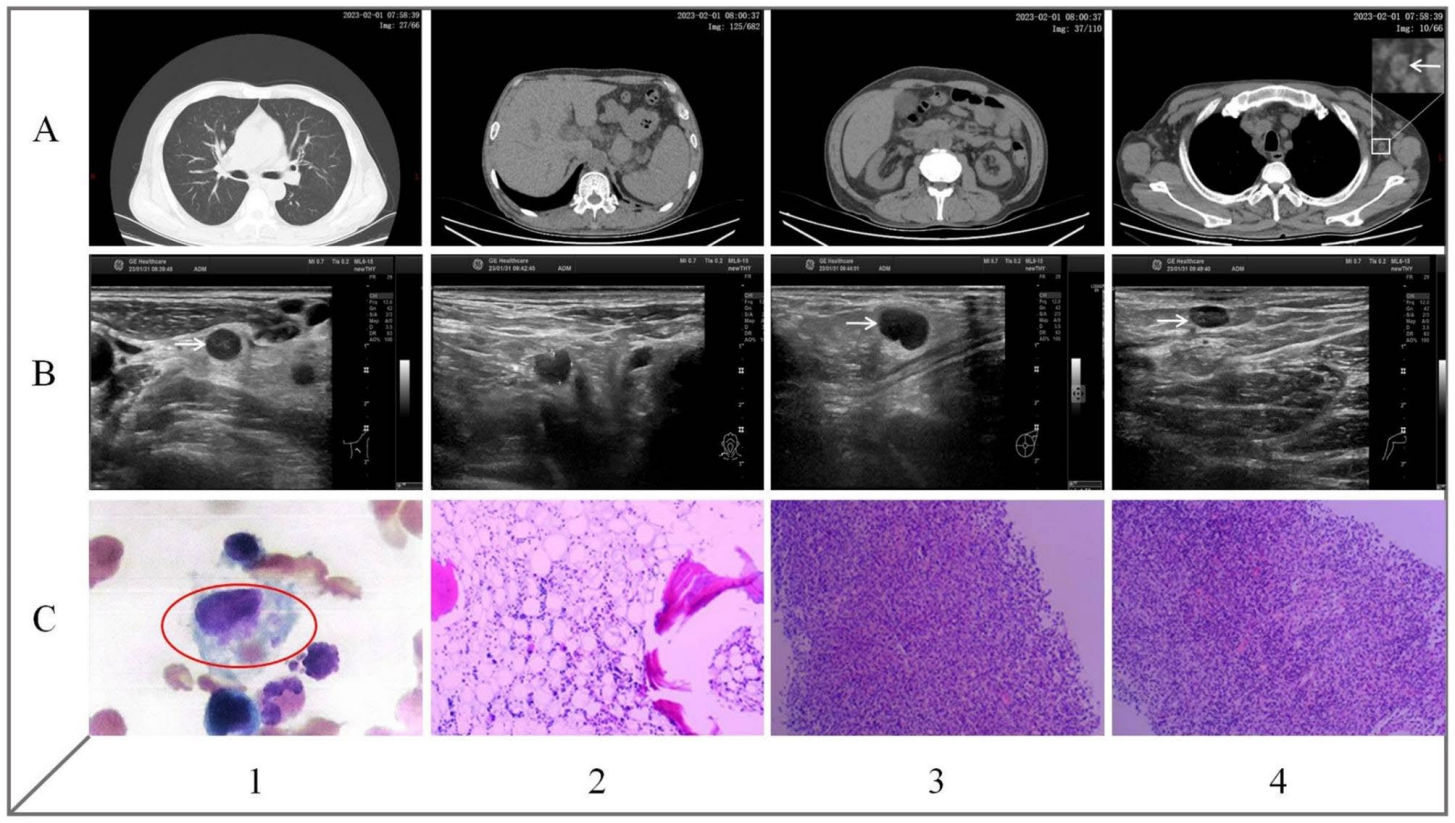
Imaging and morphologic findings of the index patient. A1-A3: Pulmonary, abdominal and pelvic CT scan shows no sign of infection, absence of hepatosplenomegaly and kidneys with slightly diminished size. A4: Mediastinal window depicts enlarged left axillary lymph node, with the ring-shape nodal necrosis (arrow). B1-B4: US image obtained at the same time as the CT scan shows a somewhat matted, hypoechoic nodal mass at the cervical, supraclavicular, axillary and inguinal sites, with an irregular perinodal border (arrow), suggesting perinodal infiltrates. C1-C2: Bone marrow aspiration and biopsy reveal trilineage hematopoiesis with hemophagocytosis (red circle). C3-C4: Lymph node biopsy finds that left cervical and axillary lymph nodes were filled with CD3^+^CD8^+^ T-lymphocytes surrounded by histiocytes in the necrotizing lesions, which was consistent with a diagnosis of KD.
